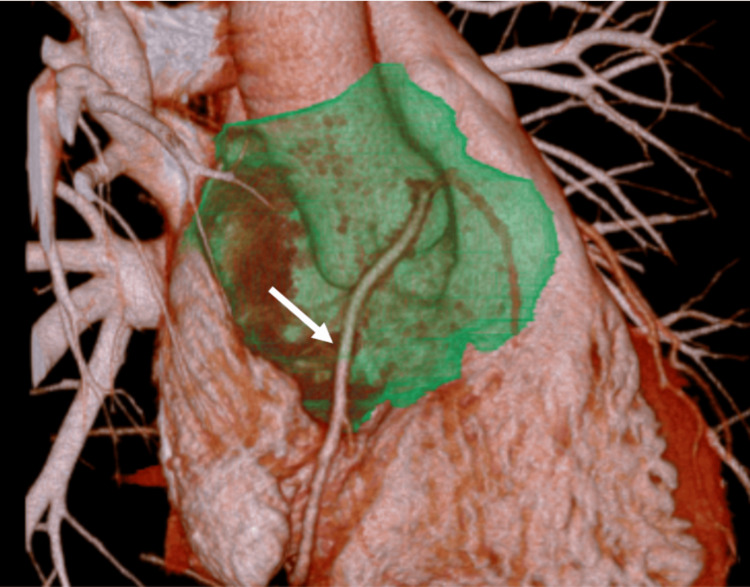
Preoperative computed tomography. The main trunk of the right coronary artery runs across the tumor (arrowhead).
